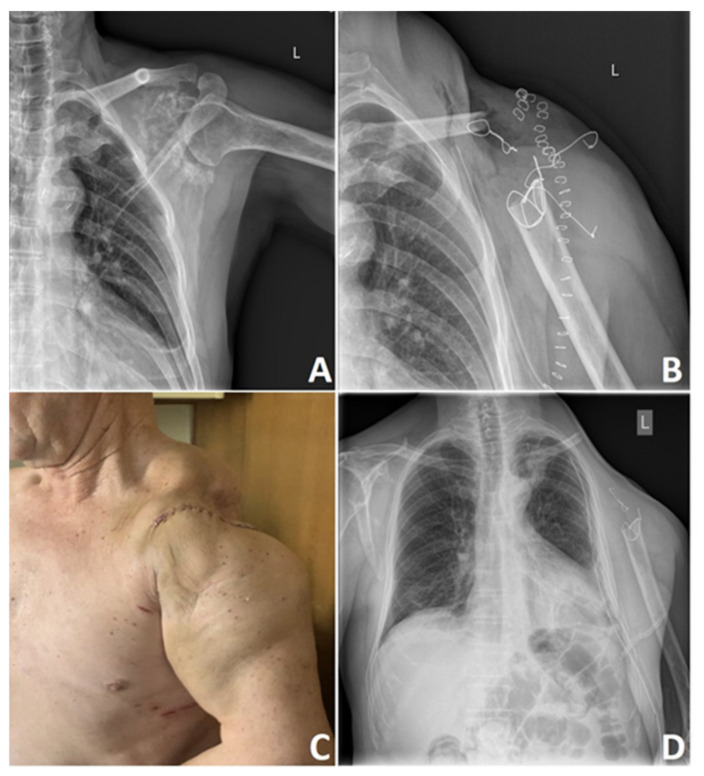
High-grade chondrosarcoma of the left scapula. Preoperative X-ray of the G3 scapular chondrosarcoma (A) and after en bloc upper interscapulothoracic humeral resection (B) This particular procedure known as Tikhoff-Linberg is a limb-sparing surgical option for tumors in and around the proximal humerus and shoulder girdle and an alternative treatment to forequarter amputation; however, is associated with poor cosmetic appearance (C) that can gradually change over the years depending on soft tissue contracture and scar tissue formation (D). Photos by Bartłomiej Szostakowski.
